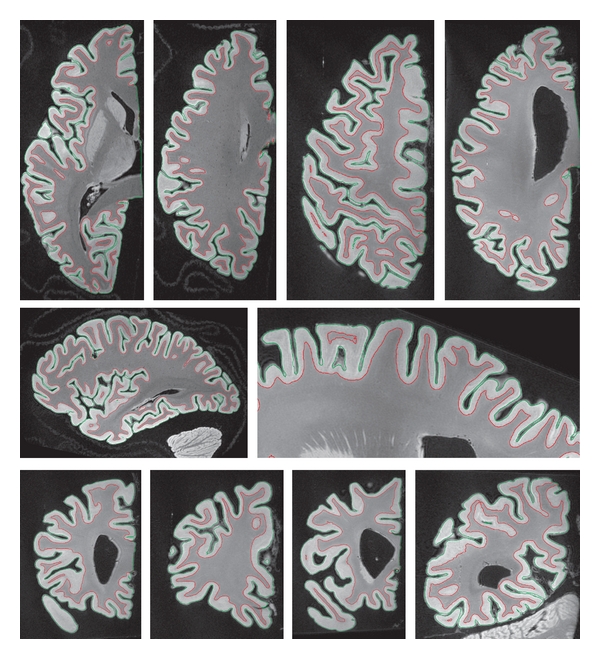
Isocontours of the zero level sets of reconstructed cortical surfaces overlaid on cross-sections of high-resolution MR images (red: the inner surface; green: the outer surface; top, middle, and bottom rows: examples of axial, sagittal, and coronal sections (not to scale), resp.).
